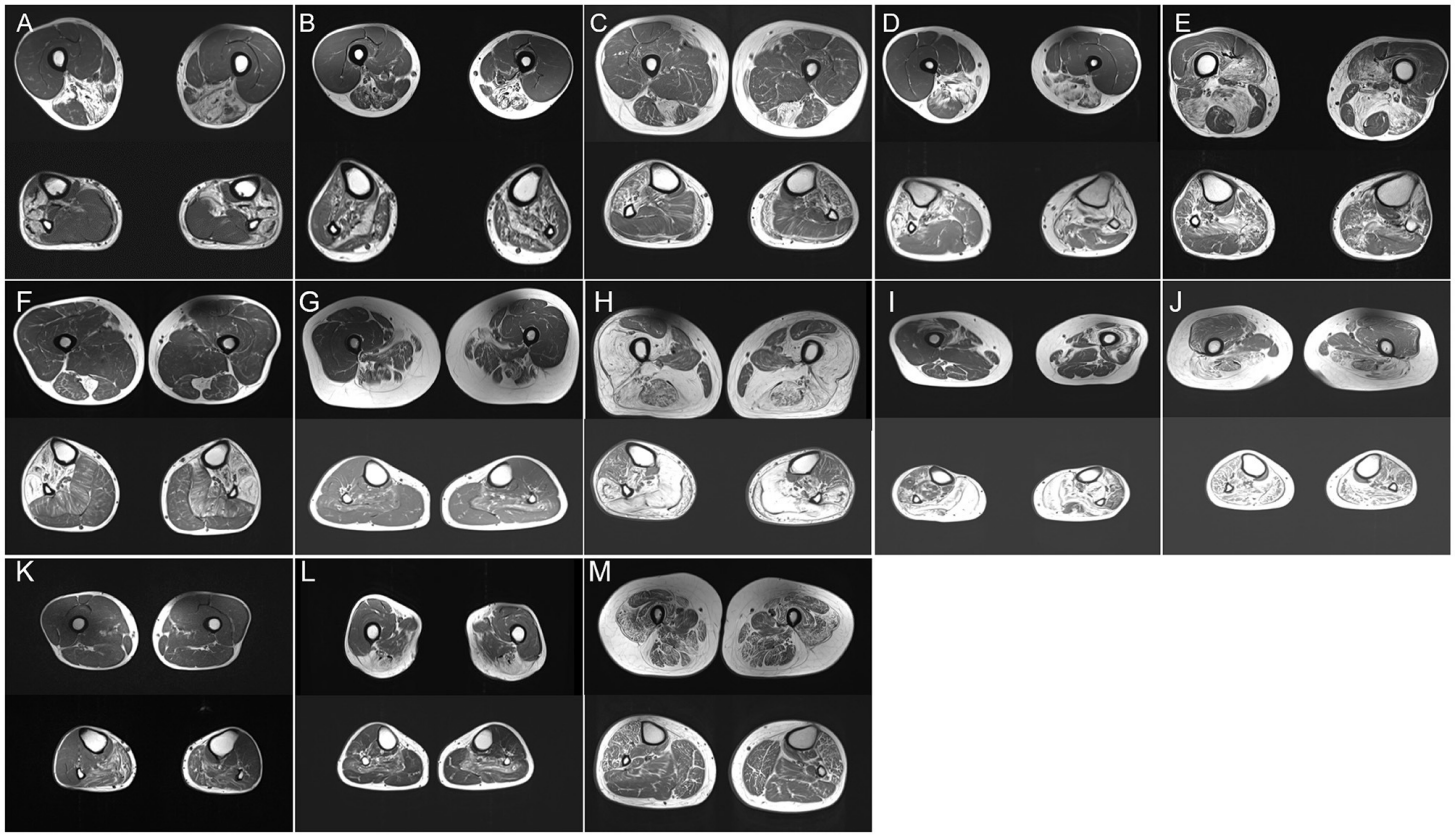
(A–M) Thigh and calf muscle MRI (T1-weighted images) of P2 (A), P12 (B), P14 (C), P15 (D), P17 I, P18 (F), P19 (G), P20 (H), P21 (I), P22 (J), P23 (K), P24 (L), and P27 (M).
